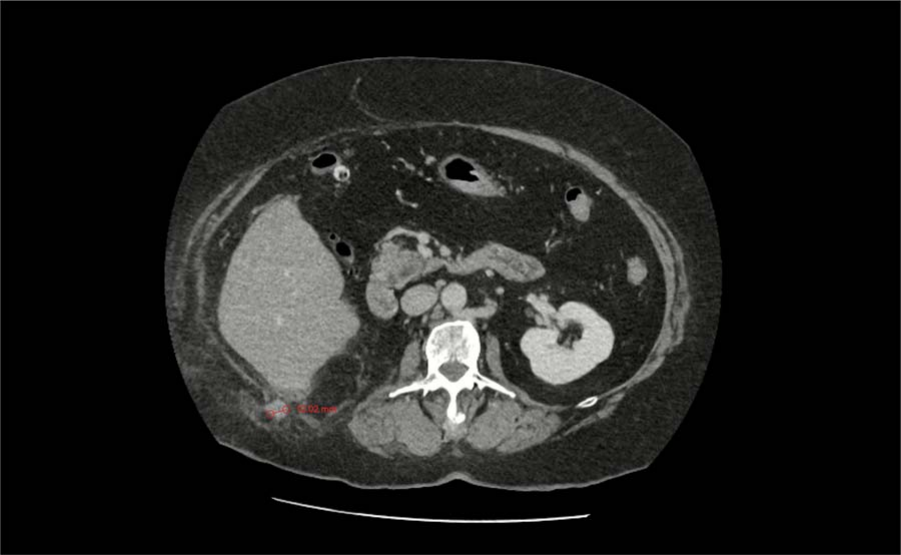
CT axial section showing a pathologically thickened appendix of 12 mm diameter (red measurement) projecting through a right posterolateral abdominal wall defect. There is also notable inflammatory fat stranding, with no evidence of perforation.
